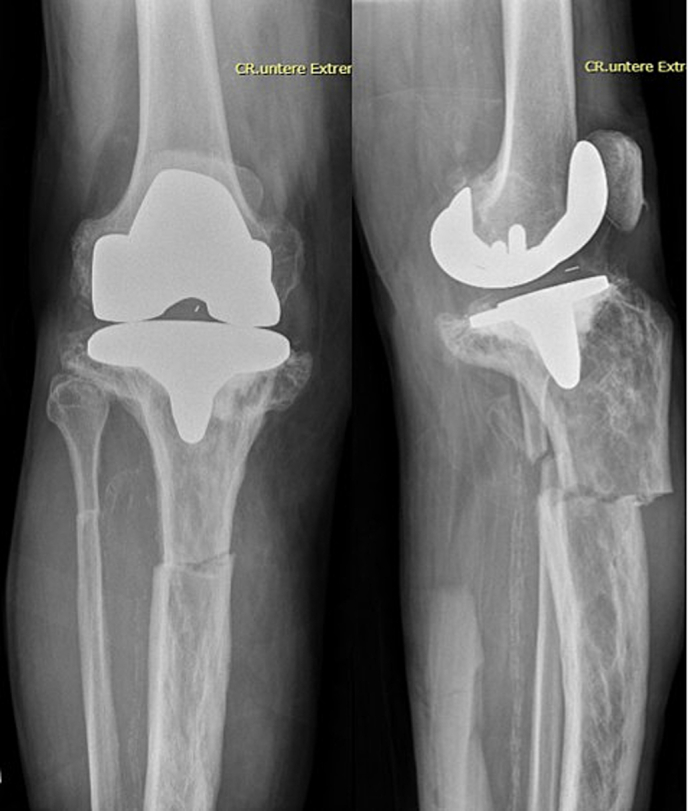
Right knee AP radiograph showing a horizontal fracture of the proximal tibial shaft, and right knee lateral radiograph revealing the fracture with an axial deviation of 9°.
